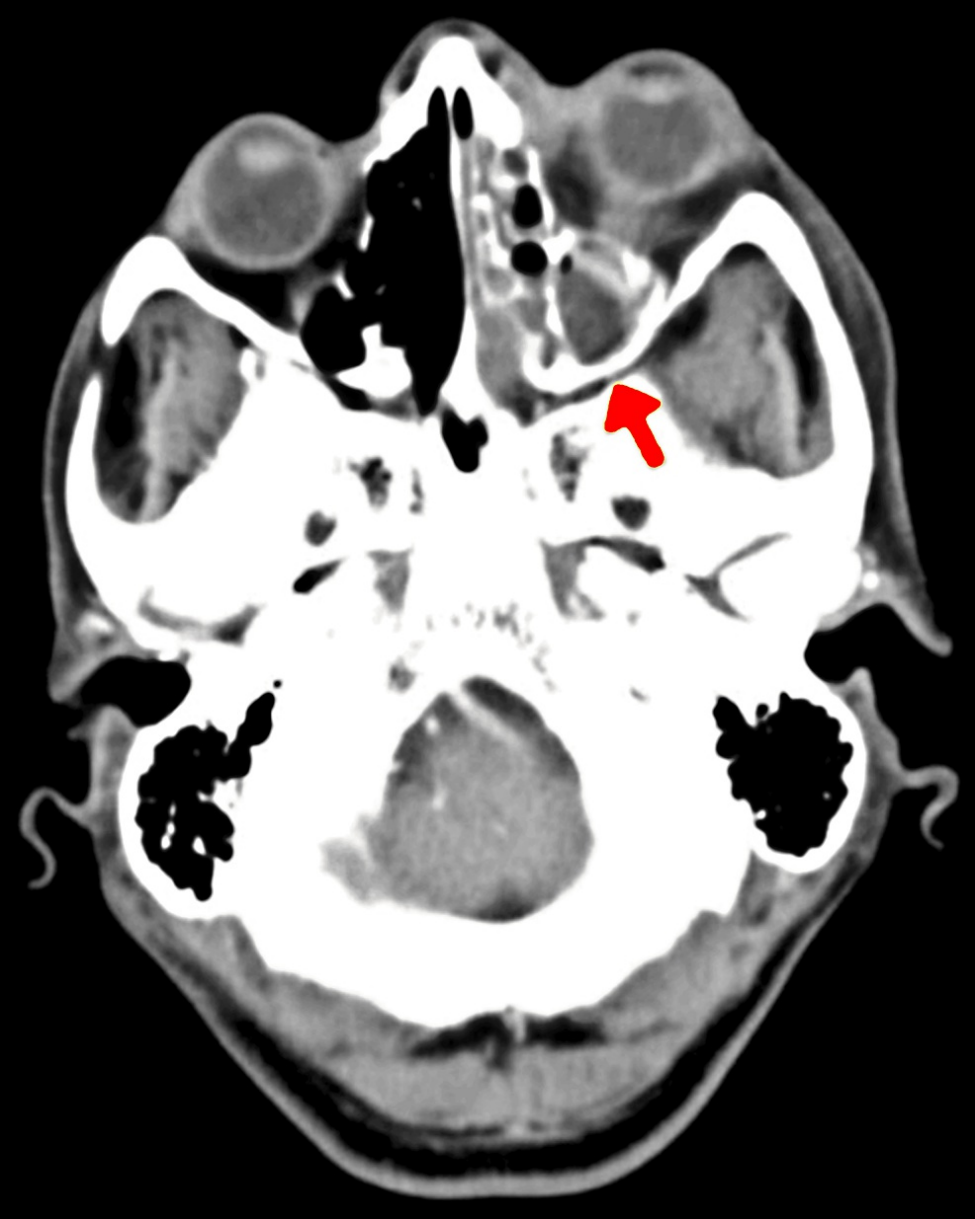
CT face plain of Case 5 shows soft tissue edematous thickening seen over the left mandible, left maxillary, and left orbital region. Right-sided deviation of nasal septum. Moderate mucosal collection in the left ethmoid and left maxillary sinus.
